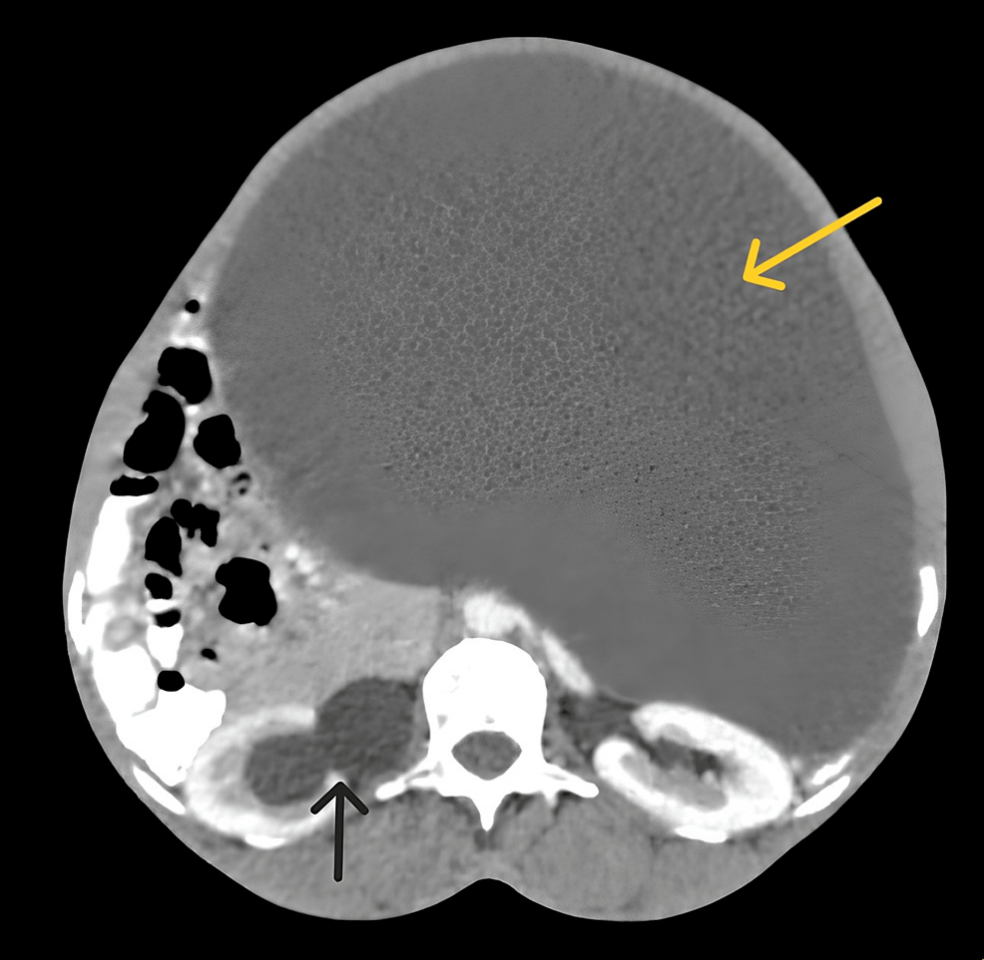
An axial section of contrast-enhanced CT scan of the abdomen showing a large cystic lobulated mass (yellow arrow) causing a mass effect on surrounding structures and hydronephrosis of the right pelvicalyceal system (black arrow).
